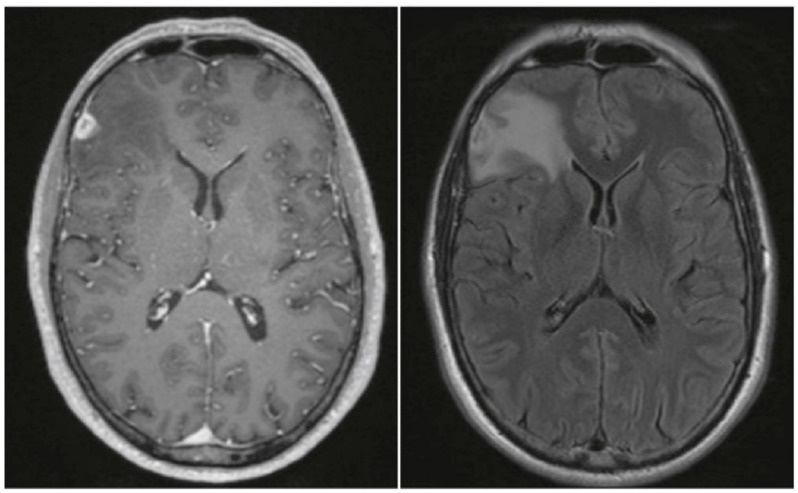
Magnetic resonance imaging of the neurocranium showed a small right frontolateral dural-based contrast-enhancing lesion measuring approximately 1.5 cm in diameter with perifocal oedema (left, T1-weighted sequence with contrast enhancement; right, fluid-attenuated inversion recovery (FLAIR) sequence).
